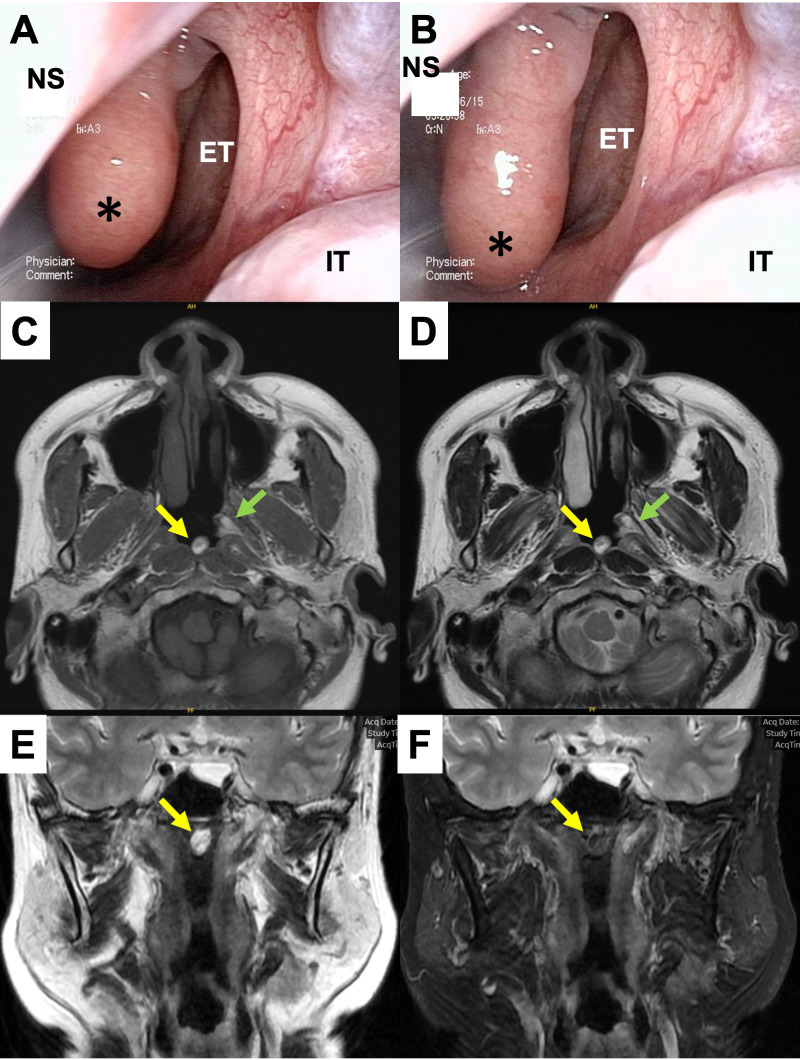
Preoperative findings. (A, B) Endoscopic findings in the left nasopharynx. A smooth-surfaced tumor originating from the nasopharyngeal superior wall was spontaneously contracting (A) and relaxing (B). *, the tumor; NS, nasal septum; ET, Eustachian tube; IT, inferior turbinate. (C–F) MRI findings at the level of the nasopharynx. The tumor showed high intensity including partial iso intensity in both T1 (C) and T2-weighed images (D, E). In a fat-suppressed image, the intensity of the tumor turned low signal (F).
